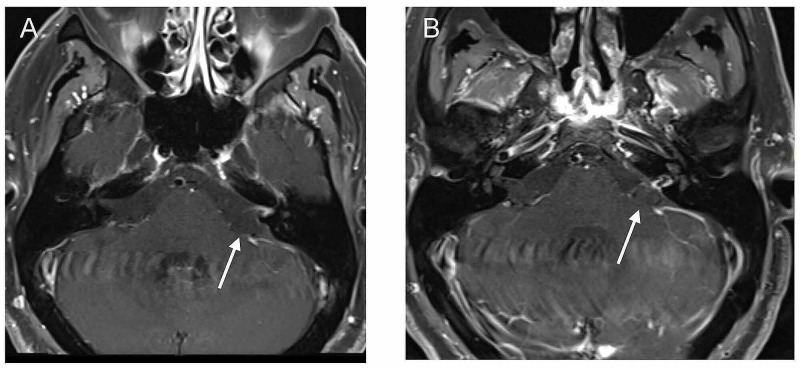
Pre-rupture MRI MRI of the brain with contrast revealed a contrast-enhancing mass at the left IAC (A), which slightly enlarged six days prior to the SAH with central non-enhancement (B). MRI, Magnetic resonance imaging; IAC, internal auditory canal; SAH, subarachnoid hemorrhage.
